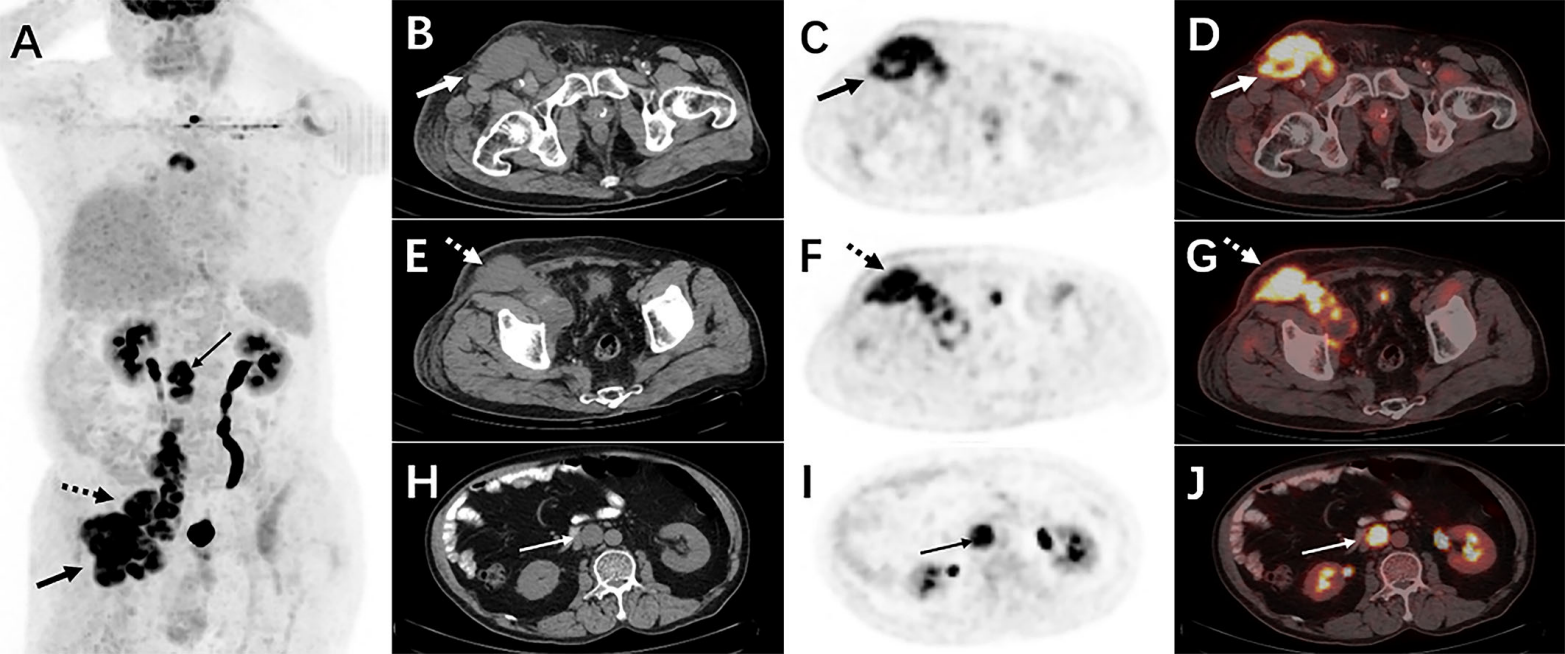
The ^18^F-FDG PET/CT imaging before treatment. The MIP picture (A) revealed hypermetabolic foci in the retroperitoneum and mediastinum (arrows). On the axial images, several low-density lesions were detected in the right inguinal region (SUVmax 12.1)(solid arrows) (B: CT, C: PET, D: fusion). The image (E: CT, F: PET, G: fusion) revealed numerous lymph nodes with abnormal metabolic confluence and a SUVmax of 9.8. (dotted arrows). Some retroperitoneal hypermetabolic foci (SUVmax 10.2) were found (thin arrows) (H: CT, I: PET, J: fusion).
